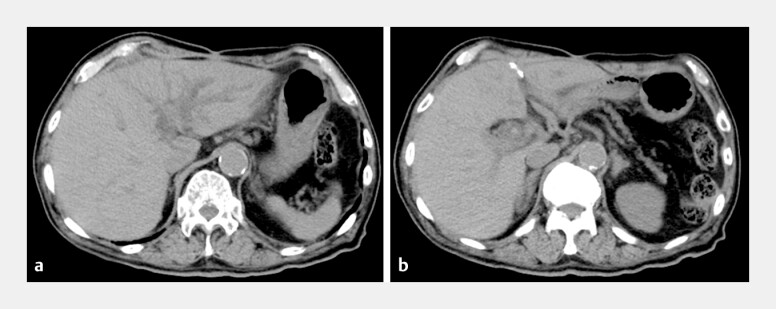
Pre-endoscopic computed tomographic scans.
